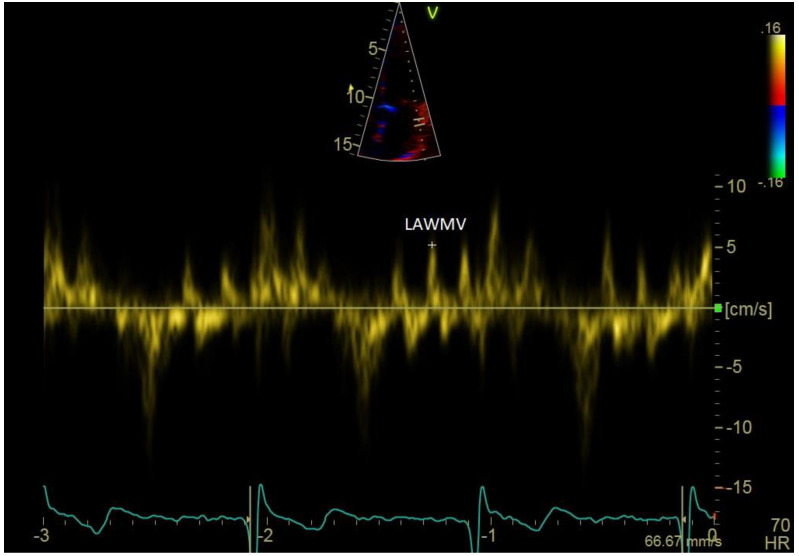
Left atrial wall motion velocity (LAWMV). LAWMV 5 cm/s. Pulsed-wave tissue Doppler in the apical four-chamber projection during atrial fibrillation.
